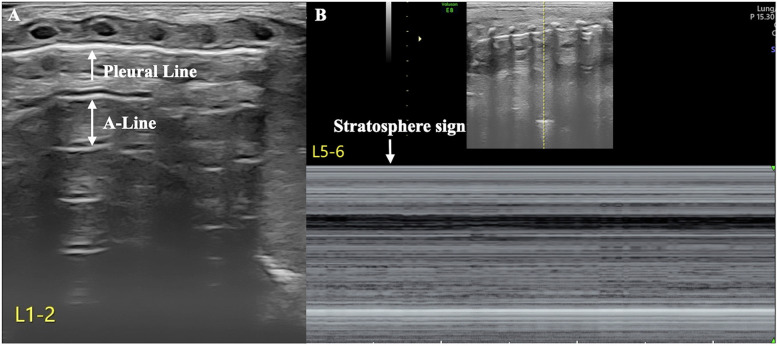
Severe pneumothorax in the left thoracic cavity. LUS revealed clear pleural lines and A-lines in the anterior chest, axilla, and posterior chest of the left lung on B-mode ultrasound. M-mode ultrasound revealed the stratosphere sign, and real-time ultrasound revealed disappearance of lung sliding (Supplementary Video S1), suggesting a large amount of pneumothorax in the left thoracic cavity (A: B-mode ultrasound, B: M-mode ultrasound).
